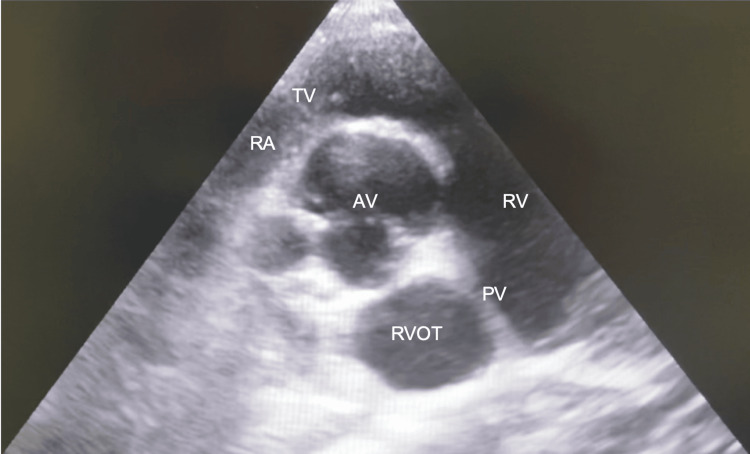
Trans-thoracic echo - parasternal aorta short-axis view highlighting a narrowed pulmonary valve AV: Aortic valve; RA: right atrium; TV: tricuspid valve; RV: right ventricle; PV: pulmonary valve; RVOT: right ventricular outflow tract
